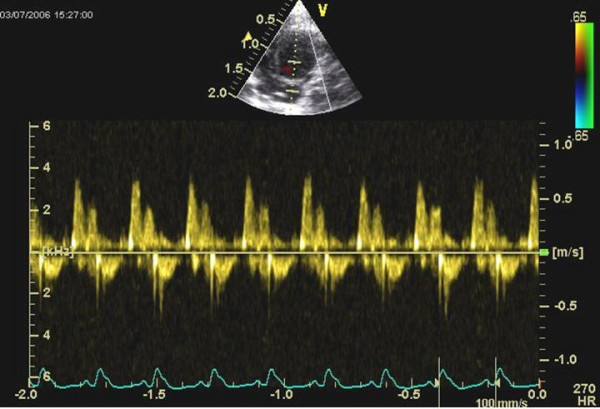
Pw-Doppler measurement of the transmitral flow velocity obtained from the apical view.
